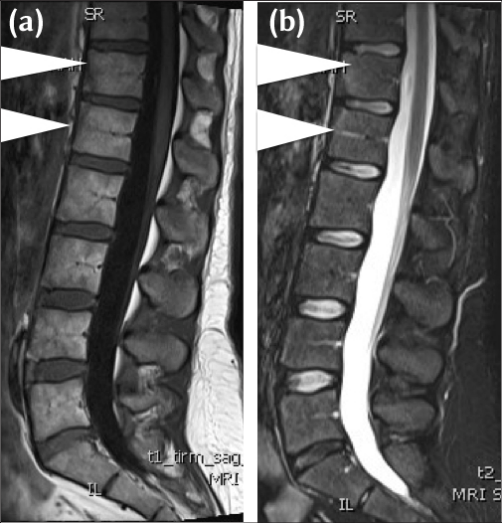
(a) 32-year-old female HLA b27 positive, with chronic low back pain and early morning stiffness. Mid sagittal T1-W MRI demonstrates triangular areas of heterogenous intermediate to low signal intensity to fat (between white arrowheads), vertebral corner lesions at T12 and LI. The features are of sclerosis and a Romanus’ Lesion on MRI. (b) 32-year-old female HLA b27 positive, with chronic low back pain and early morning stiffness. Mid sagittal STIR MRI demonstrates corresponding triangular areas of low signal intensity (between arrowheads) at T12/L1. The features are of sclerosis and a Romanus’ Lesion on MRI.
